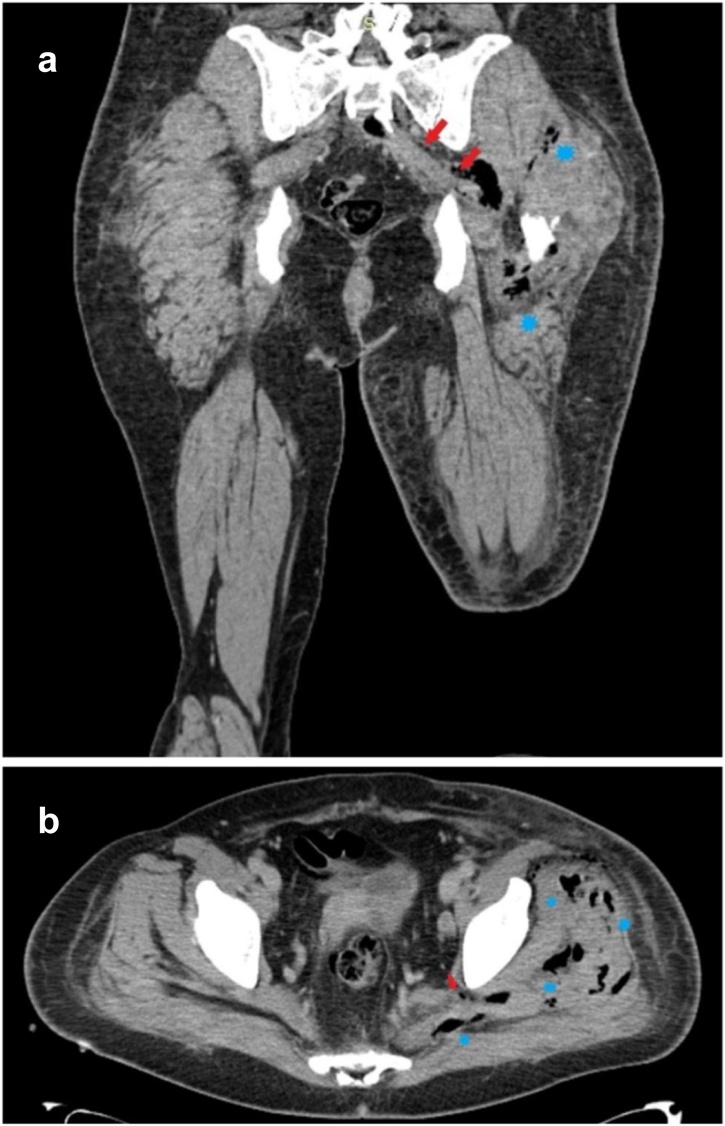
Coronal (a) and axial (b) CT images of inferior part of pelvis and gluteal region. *Blue stars* mark free gas in soft tissues and muscles around left hip area (phlegmon). *Red arrows* show continuity of gas interpositions from pelvis to soft tissues through the greater sciatic notch.
